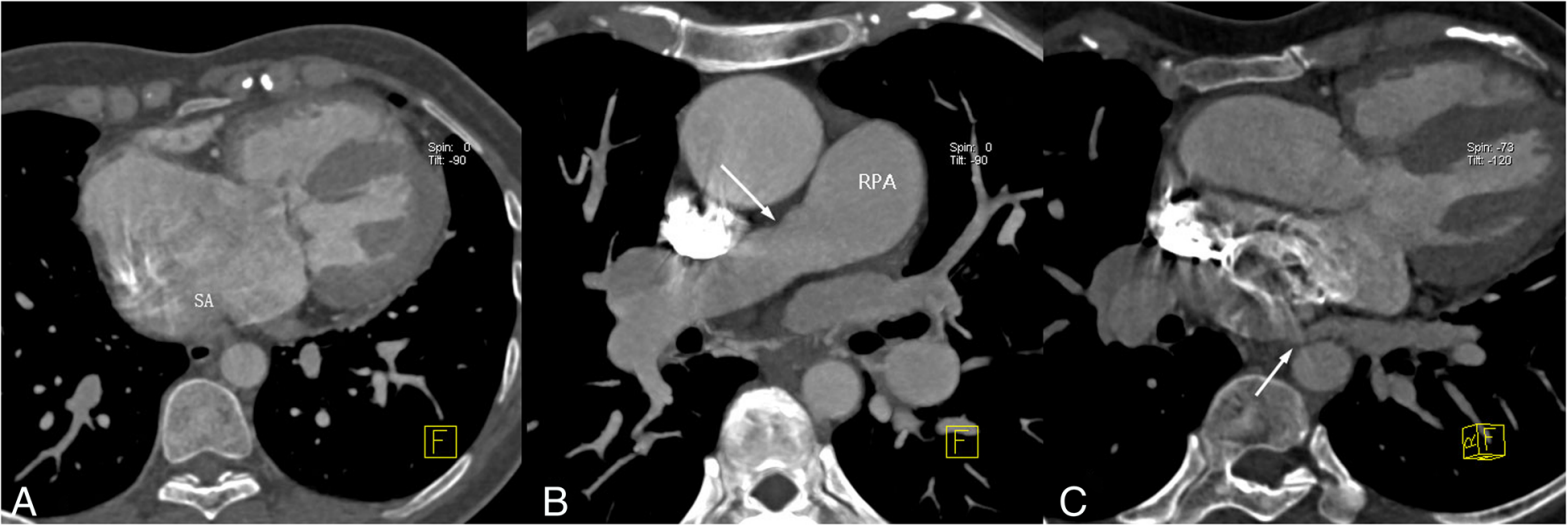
Computed tomography angiography. a, b Axial CT imaging revealed an enlarged single atrium (SA), and mild stenosis (*arrow*) in the RPA. c Axial CTA imaging showed a narrow ostial (*arrow*) by which the left inferior pulmonary vein entered into the left side of a common chamber
